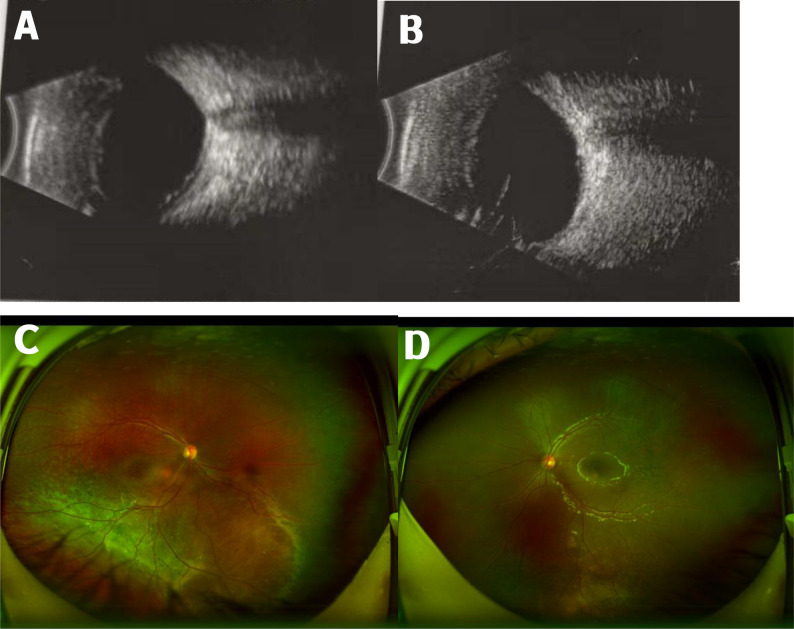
Examinations after two months. A and B. Ultrasound B-scan in both eyes showed retinal detachment C. SLO image in right eye showed area of retinal detachment in the inferior part reduced. D. The SLO image in the left eye showed a small area of retinal detachment in the inferior quadrant
